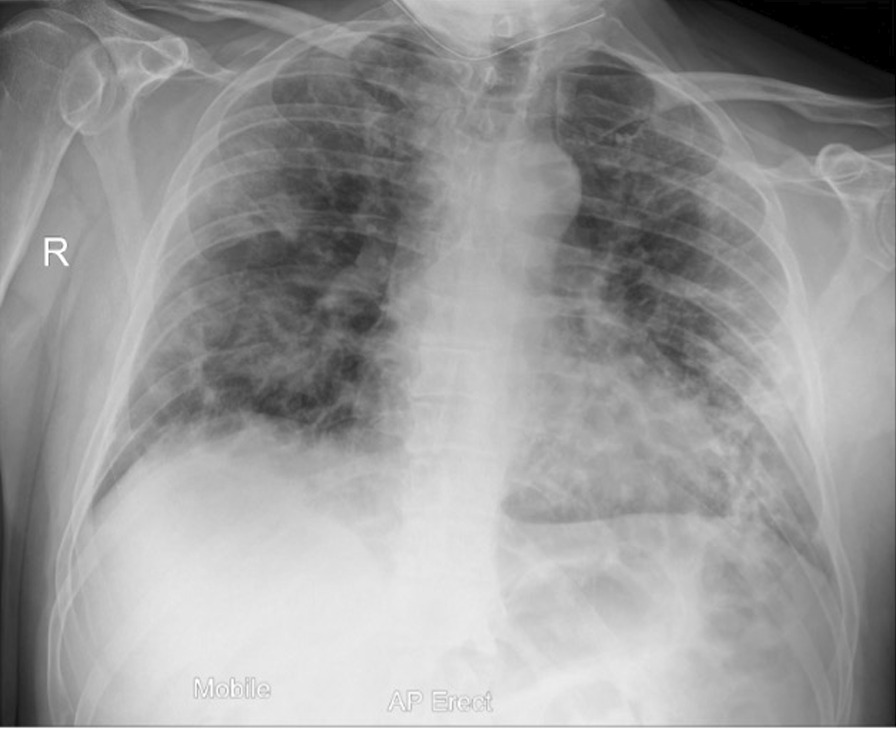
Chest x-ray on initial admission to hospital: Extensive multifocal opacities throughout lungs in keeping with COVID-19 pneumonitis
